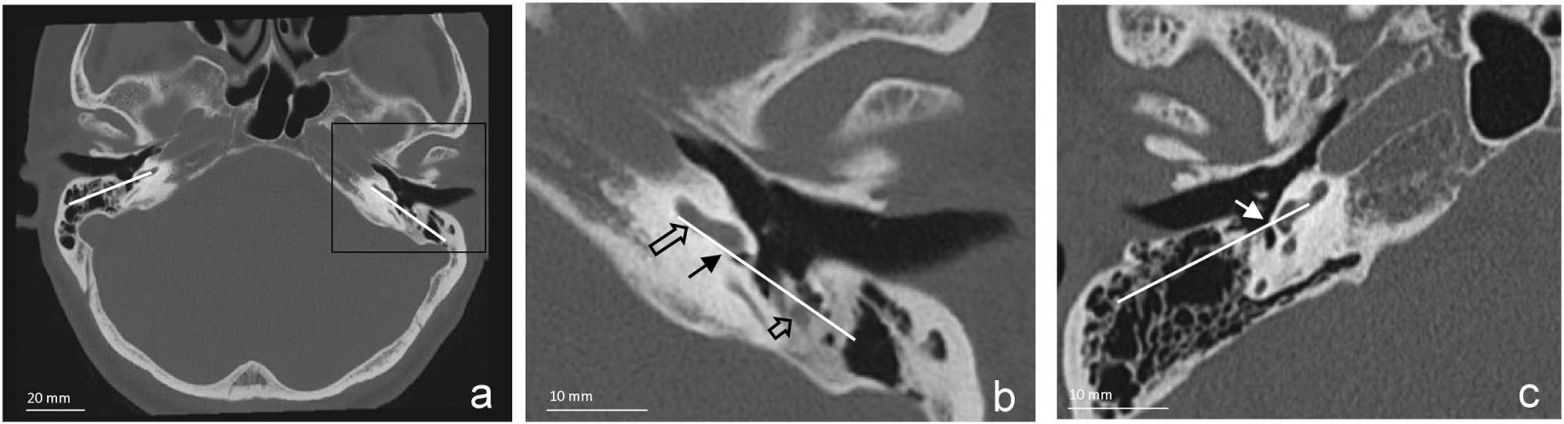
a Prediction line drawn on the axial high resolution scan of both temporal bones. b Close up view of the prediction line. The prediction line was drawn on the preoperative axial high resolution CT scans between the anterolateral mastoid facial nerve and the lower side of the basal turn of the cochlea. The intersection point lies on the posteromedial side of the round window membrane in this example. Large unfilled arrow = lower side of basal turn, filled black arrow = posteromedial intersection point, small unfilled arrow = facial nerve. c Example of an intersection point on the anterolateral side of the RWM. White arrow = anterolateral intersection point
